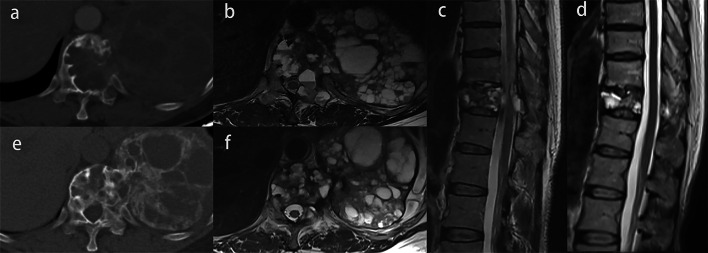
Image of a giant cell tumor of T11 in a 50-year-old woman. a, e Axial CT scans before and after denosumab treatment. Complete peripheral rim and bone formation inside the mass over 50% of the transverse session are observed (grade 3). b, f Axial MR images before and after denosumab treatment. The maximum diameter of the tumor and the proportion of the area of the spinal canal occupied by the tumors are smaller. c, d Sagittal MR images before and after denosumab treatment. The spinal cord compression completely disappears
